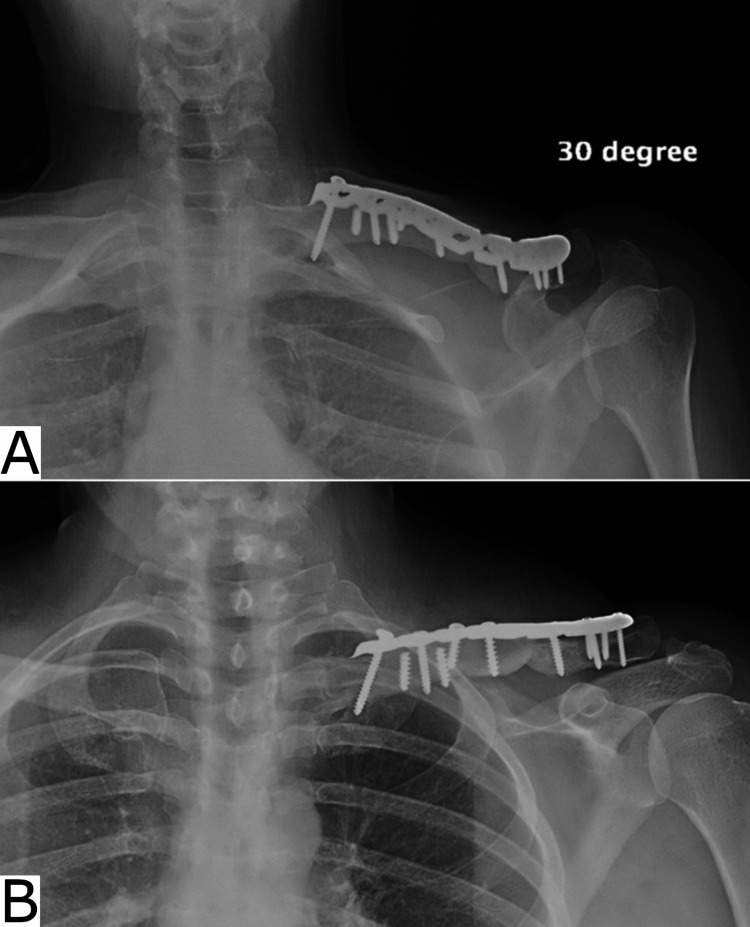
Eight-month postoperative radiograph taken from 30 degrees below the clavicle (panel A) and direct anteroposterior (panel B) showing bony bridging and complete healing of the graft.
